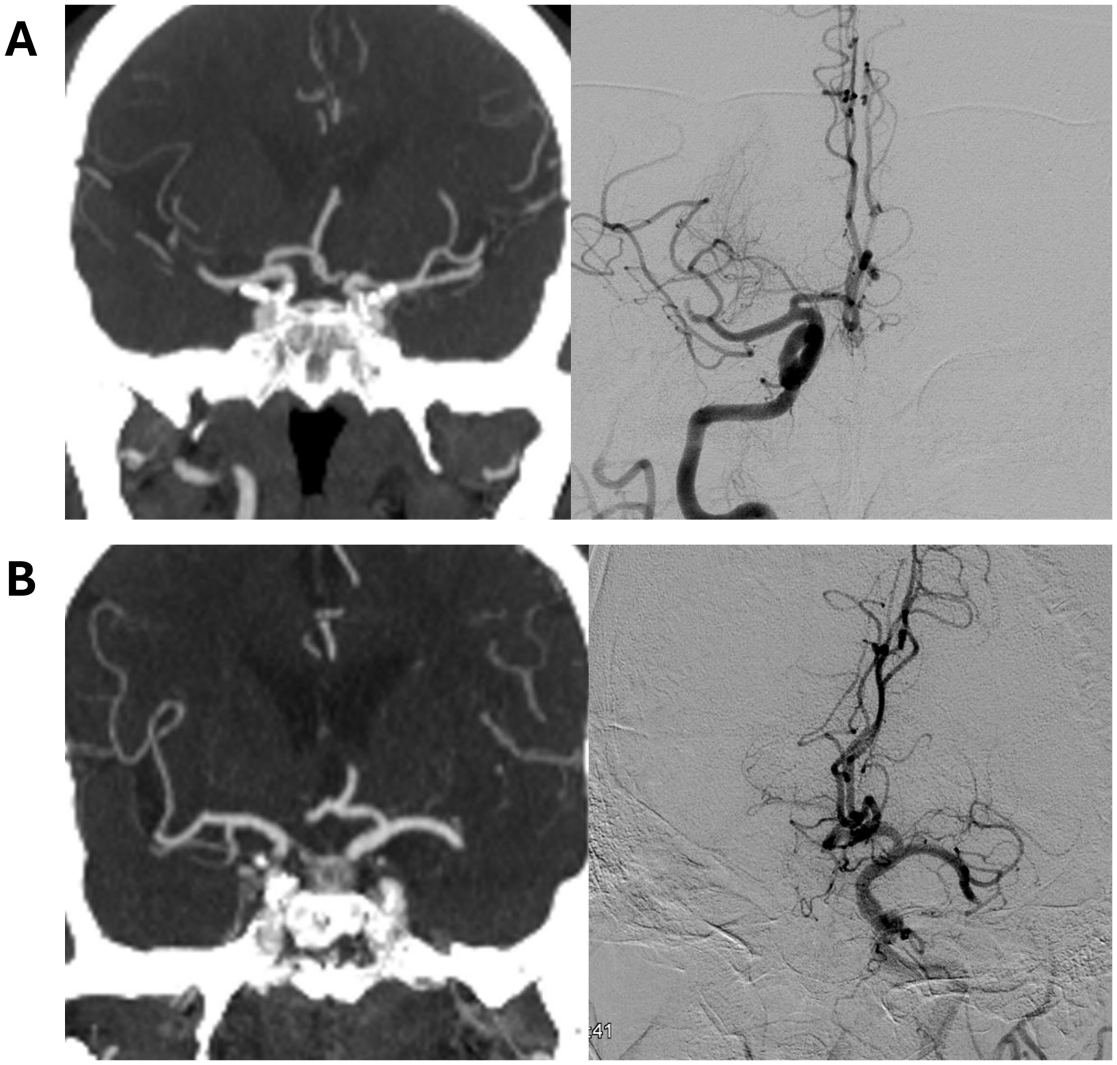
Definition of thrombus migration by discrepancy between CT angiography and digital subtraction angiography. Representative examples show (A) intersegmental thrombus migration from the right MCA M1 segment to the M2 segment and (B) intrasegmental thrombus migration in the left MCA.
